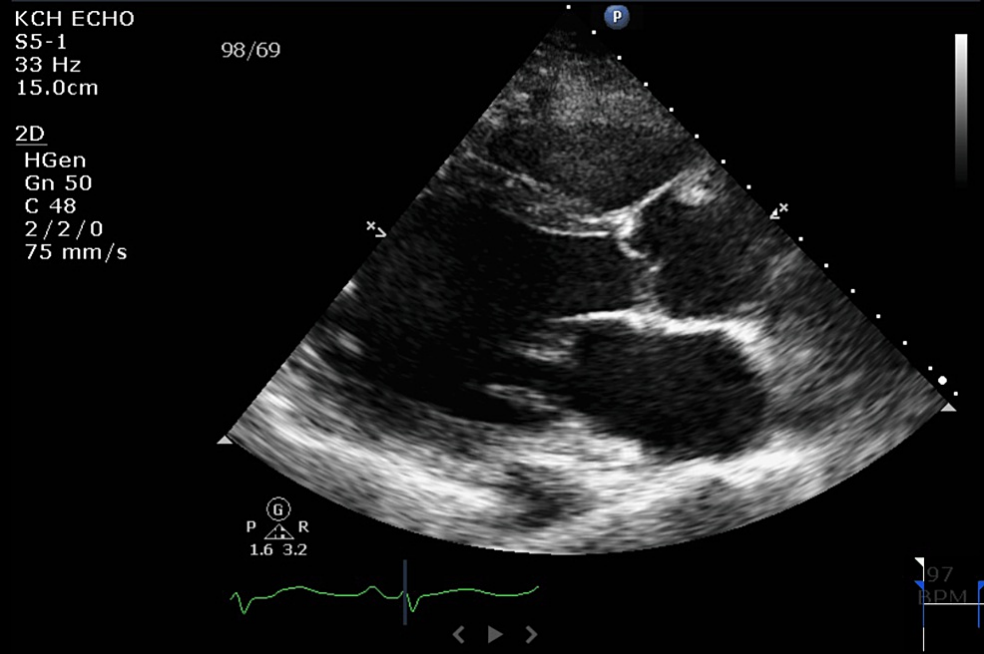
TTE on 12/6/2022 showing the end-systole phase with LVEF 5% TTE: transthoracic echocardiography; LVEF: left ventricular ejection fraction
